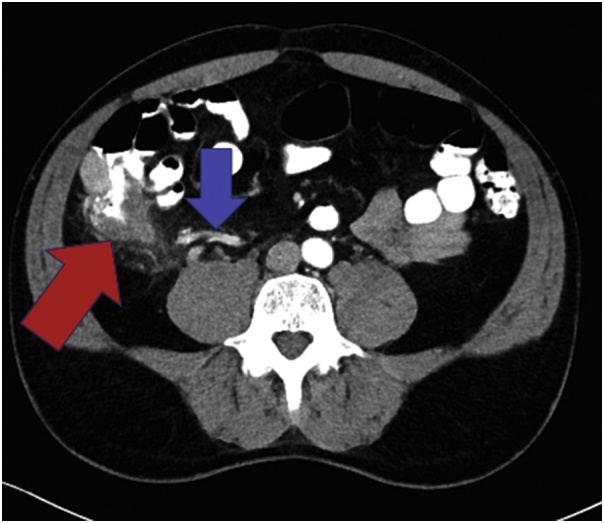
Abdominal CT image at admission with IV and oral contrast. Multiple contiguous axial images of the abdomen and pelvis were obtained following the administration of intravenous and oral contrast. The appendix is dilated to 1.8 cm with significant periappendiceal stranding and appendiceal wall thickening (blue arrow). Also in the right lower quadrant, either adjacent to or within the wall of the cecum, is a bowel mass measuring 2.3 × 1.9 × 2.3 cm, concerning for a malignancy (demonstrated by the red arrow).
